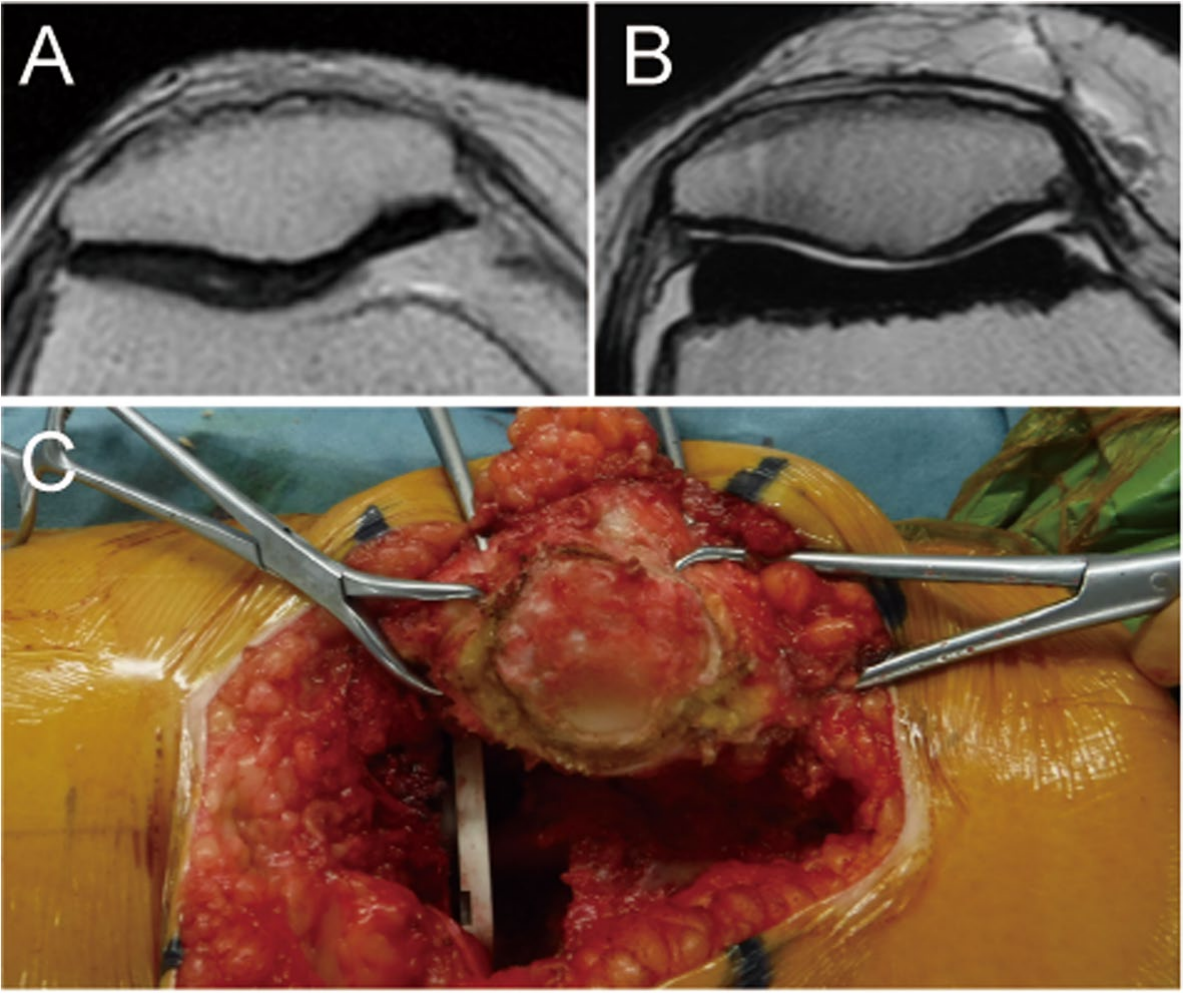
The patient underwent conversion to patellar resurfacing after non-patellar resurfacing TKA. A MRI before surgery (B) MRI after non-patella resurfacing TKA; the patella thickness is decreased compared to the thickness before TKA (C) Intraoperative view of the patellar cartilage reveals excessive articular cartilage wear of the patella in patients undergoing non-patella resurfacing total knee arthroplasty. TKA, total knee arthroplasty; MRI, magnetic resonance imaging
